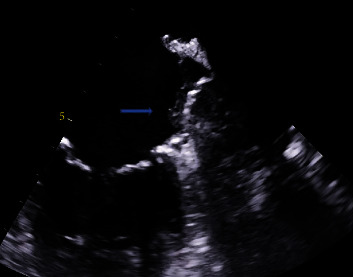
Repeat transesophageal echocardiogram showing a decrease in clot formation on a WATCHMAN FLX™ device within the left atrial appendage.
